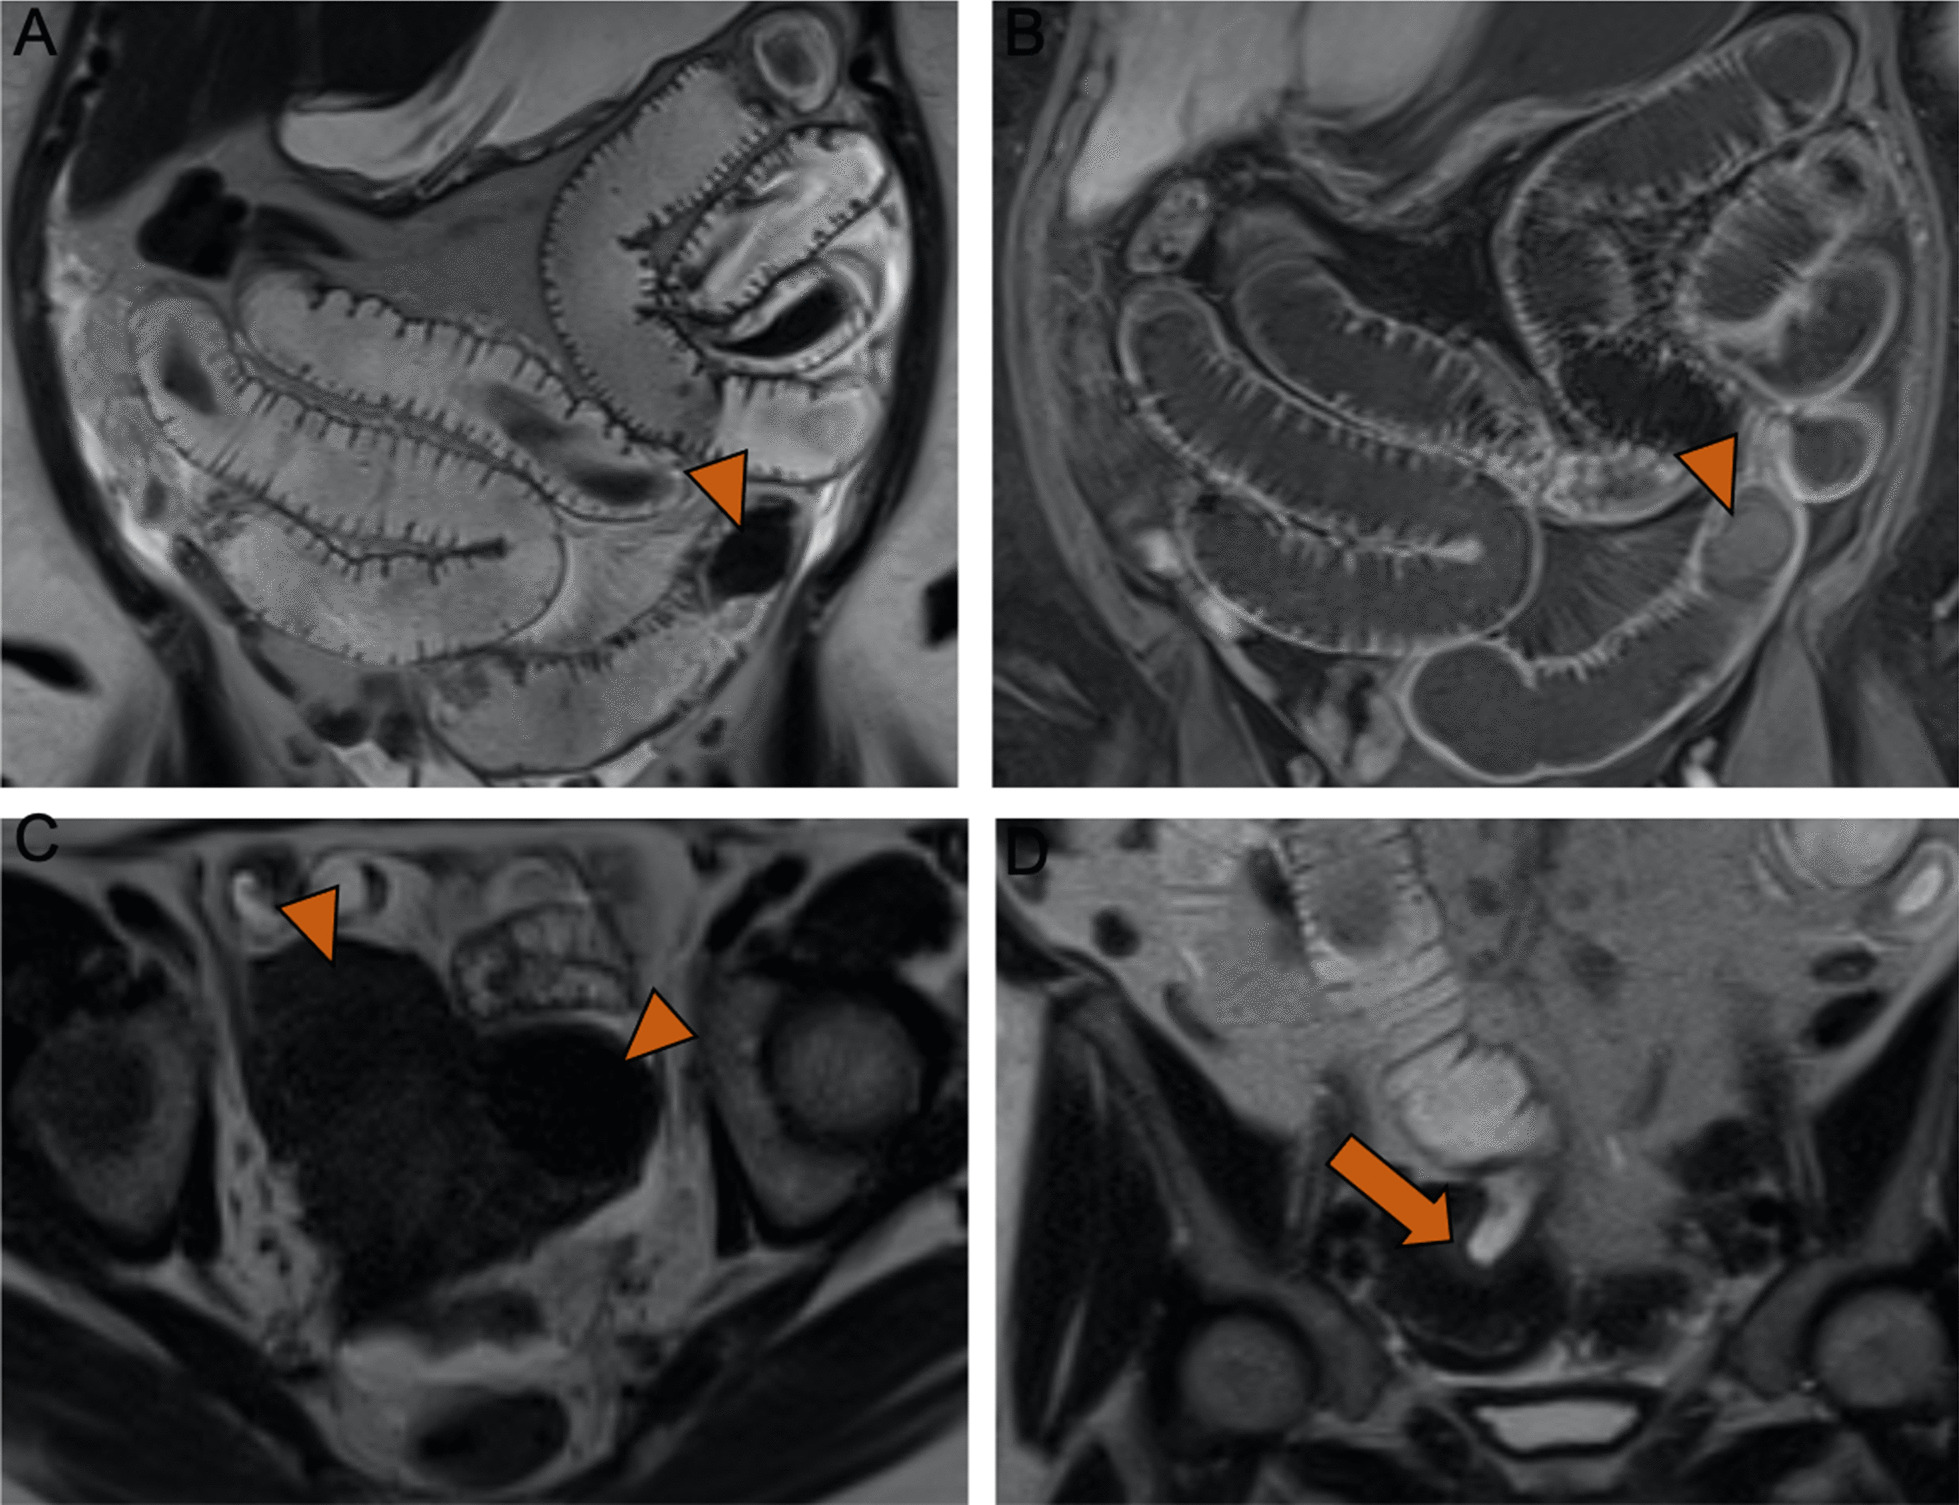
Magnetic resonance imaging with coronal T2-weighted (A, C), axial T2-weighted (B), and coronal contrast-enhanced T1-weighted (D) images. A Small bowel obstruction is again noted with an intraluminal T2-dark lesion within the ileum (arrowhead). B Similar T2-dark lesions (arrowheads) are noted in the uterus, in keeping with uterine leiomyomas. C, D A uteroenteric fistula (arrow); the uterine fundus and the ileum served as a conduit for migration of a leiomyoma into the ileum, leading to the small bowel obstruction
